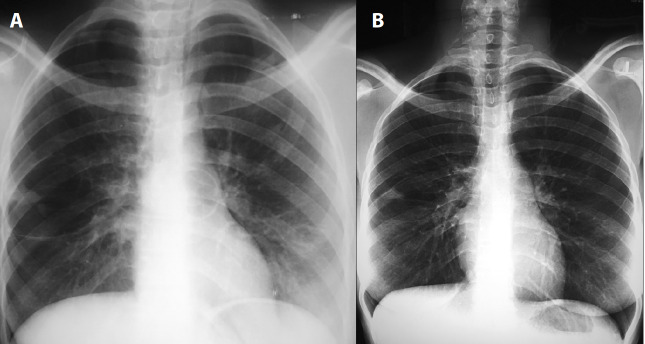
(A) Resolving lung opacities 3 months after starting fluconazole therapy. (B) Normal chest X-ray 6 months after starting treatment.
